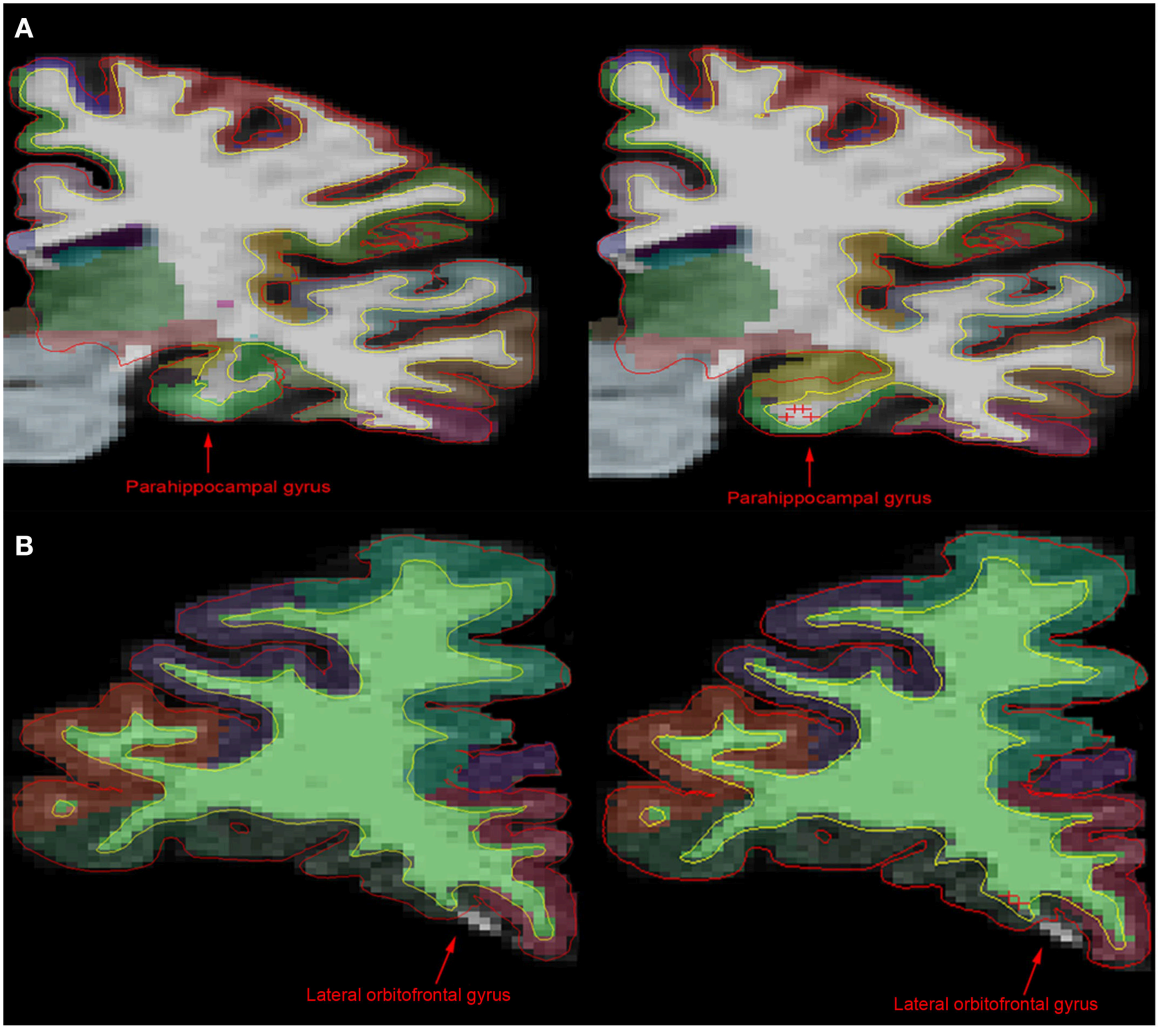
Comparison of MR images before and after manual intervention. (A) In comparison with the unedited 1.5T image (left), the manually edited brain image (right) shows a more accurate portrayal of the parahippocampal gyrus, the hippocampus and the white matter boundary. (B) However, in the 3T brain images, there is little difference between the unedited (left) and the manually edited (right) images. The manual intervention implemented in the 3T brain was intended to include white matter and gray matter incorrectly being excluded from the lateral orbitofrontal gyrus area. Control points on this slice in addition to edits on anterior and posterior brain slices had no significant effect on the exclusion. This shows that although control points can have an effect on white matter and pial surface, as well as cortical parcellation, it is inconsistent.
